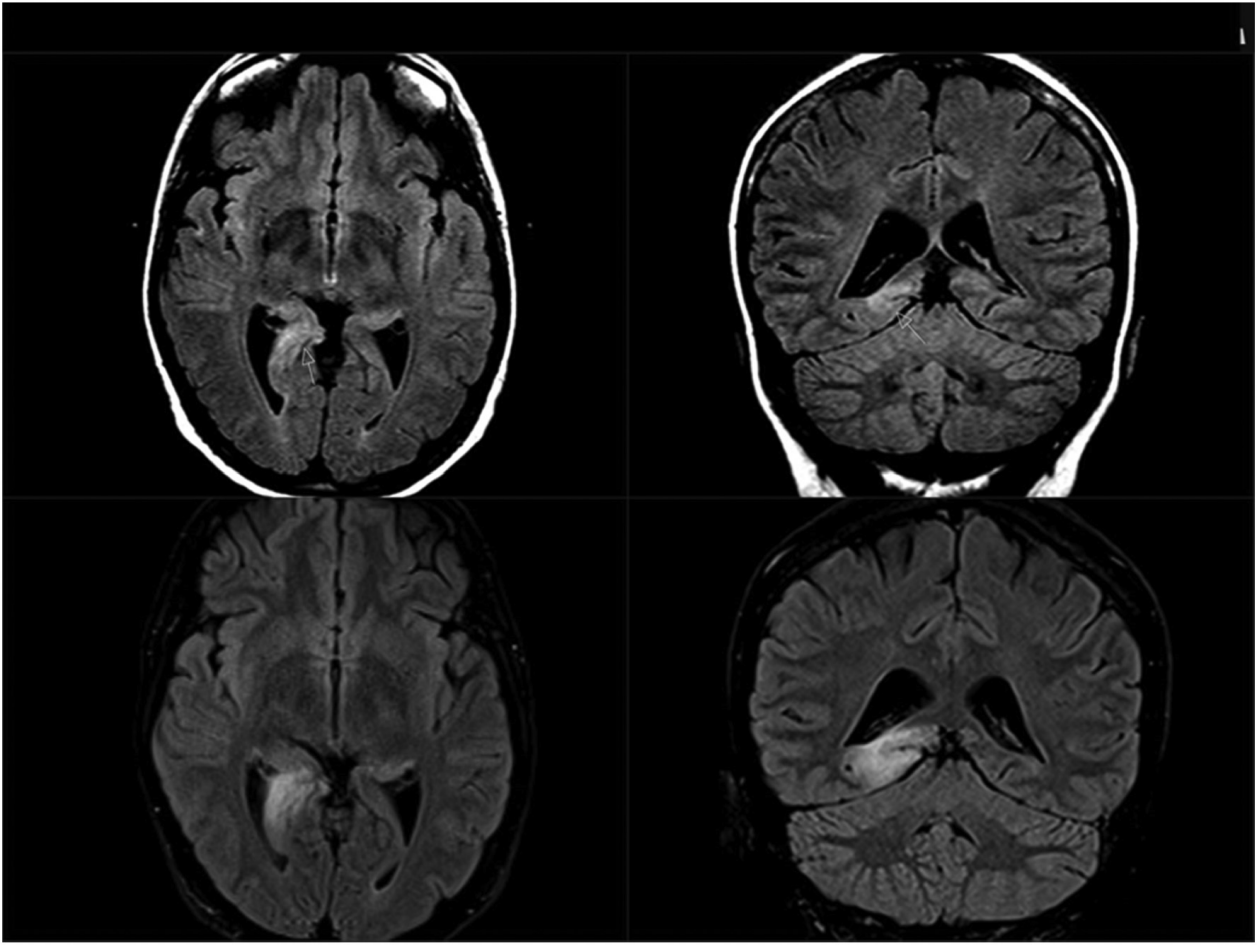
MRI findings. FLAIR images (axial plane, left; coronal plane, right). Upper: Prior to serial seizures with complex hallucinations. Lesion in the posterior part of the right parahippocampal gyrus and in the hippocampal tail. Lower: During serial seizures. Substantial volume increase of the lesion with extension toward the occipital cortex.
